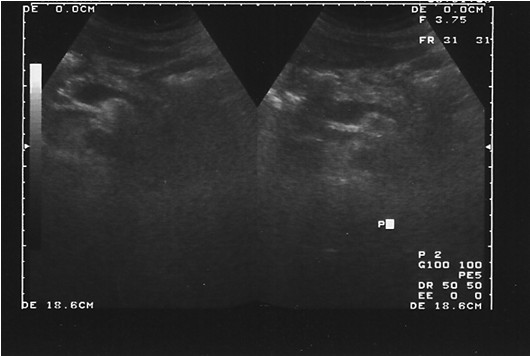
Abdominal ultrasound showing the normal pancreas six months after the episode.
